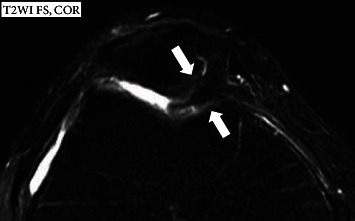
MRI showed a thickening of the medial plica protruding far into the medial patellofemoral joint (PFJ).
